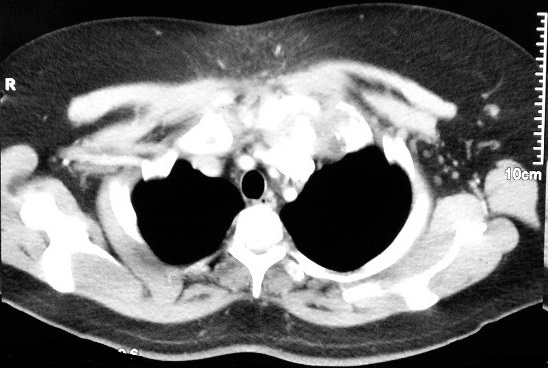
CT scan showing lobulated collection in the subcutaneous tissue of the left lower neck extending into left sternoclavicular and first sternochondral joint
